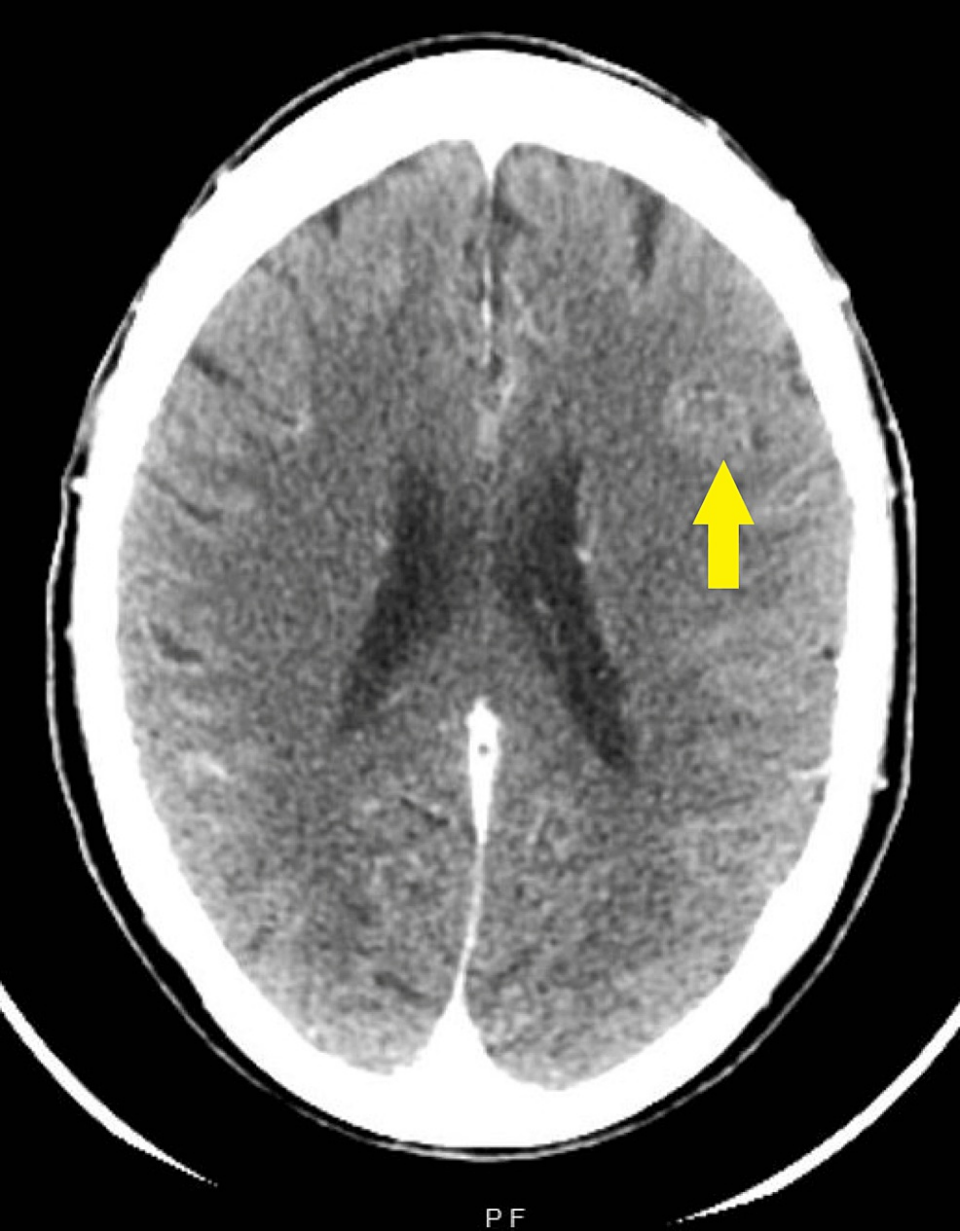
Post-contrast head CT. Arrow is pointing to the questionable left frontal lobe lesion that seemed to enhance with contrast.
